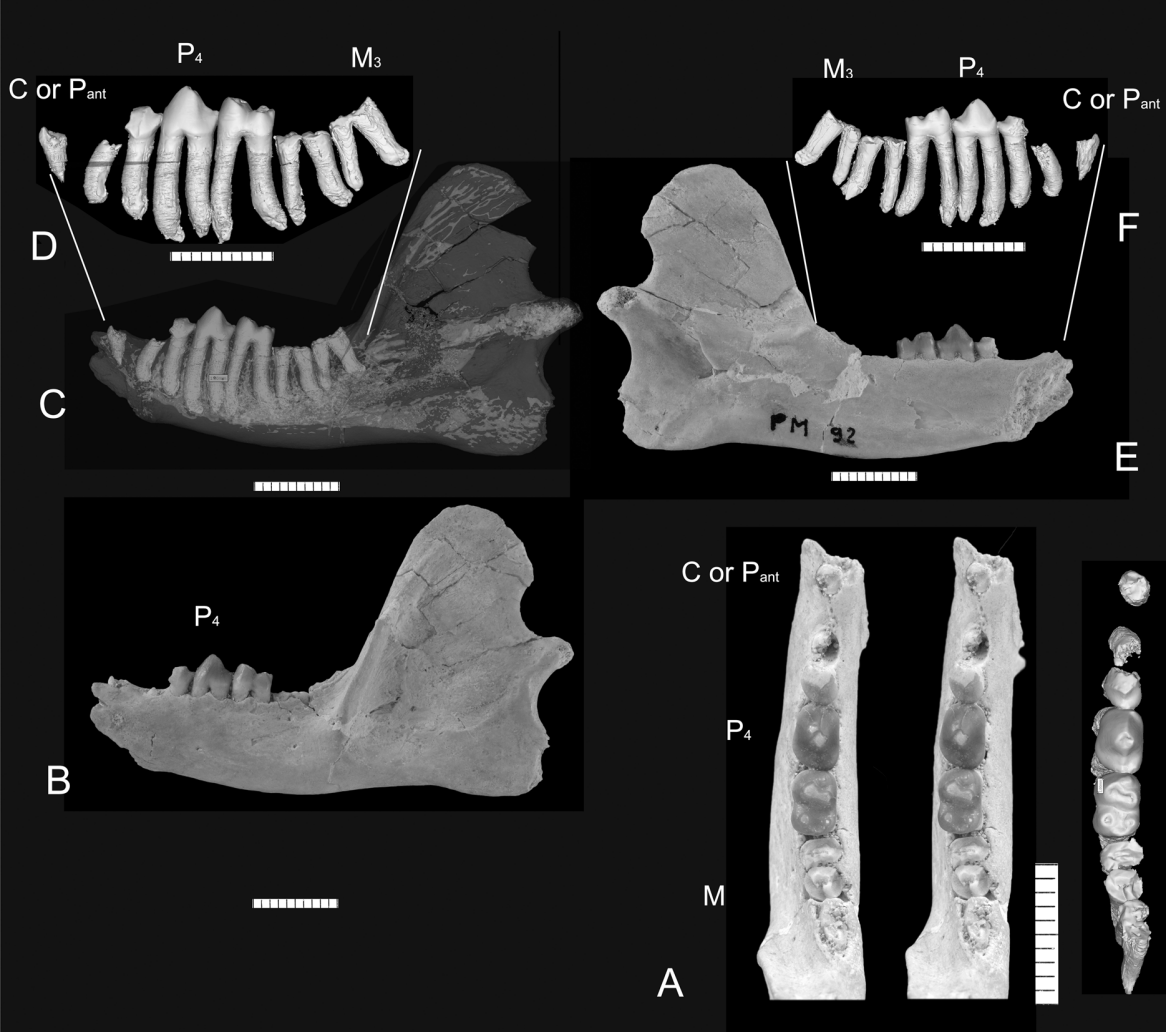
Abdounodus hamdii*, Selandian (Phosphate level IIa) of the Ouled Abdoun Basin, Morocco. MNHN.F PM92, left dentary bearing roots of M_3_ and M_2_, crown of M_1_, P_4_, P_3_ (damaged) and root of P_2_ or P_1_ or C_1_. (A) stereophotographic pair in occlusal view and 3D model of the isolated teeth in occlusal view reconstructed from the CT scans; (B) Labial view; (C) Transparent 3D model in labial view reconstructed from the CT scans and showing the roots of the teeth; (D) 3D model of the isolated teeth in labial view, reconstructed from CT scans. (E-F) Same in lingual view. Scale bar = 10 mm.
